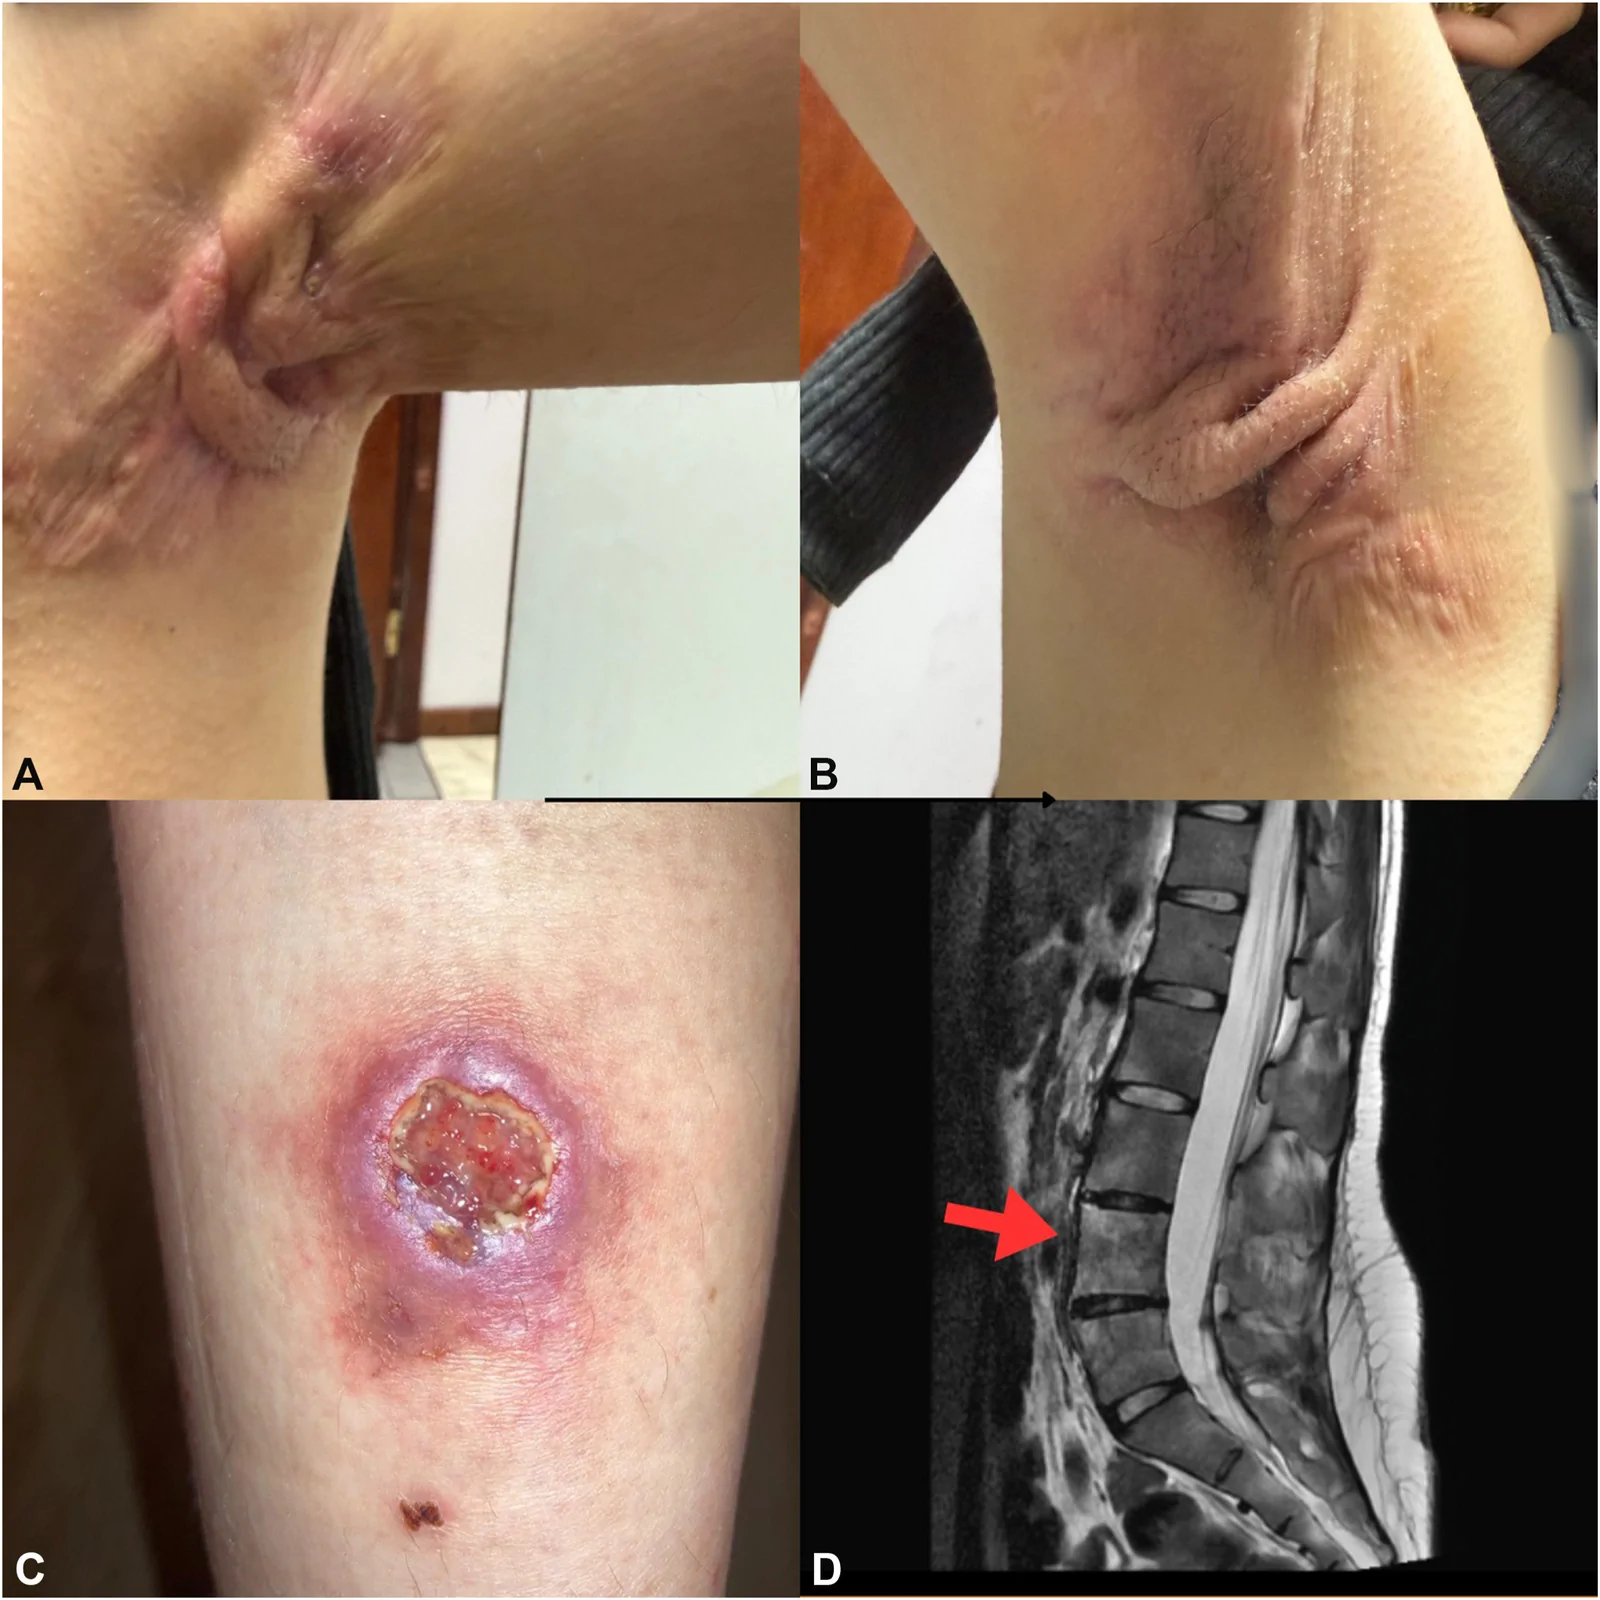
Hidradenitis suppurativa Hurley Stage III on left (A) and right (B) axilla, well-controlled, without active draining sinus tracts or cysts, 4 months after treatment with secukinumab in 2022. Pyoderma gangrenosum involving the right lower extremity in 2025 following treatment with secukinumab 300 mg subcutaneously every 2 weeks for hidradenitis suppurativa (C). D, Sagittal lumbar MRI showing diffuse increased T2 signal with inflammatory changes at L3-L4 and L4-L5 (D). The *red arrow* indicates the diffuse increased T2 signal with inflammatory changes seen on the MRI. *MRI*, Magnetic resonance imaging.
